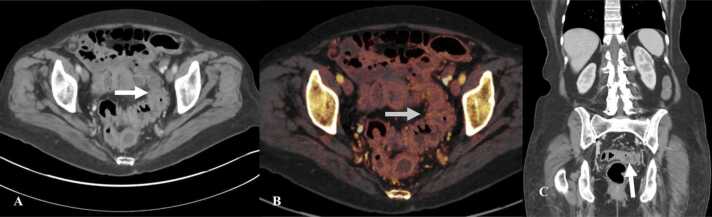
Immune-mediated colitis in a 73-year-old woman with renal cell cancer on Nivolumab. A. Contast-enhanced axial CT of the abdomen and pelvis shows wall thickening and enhancement of the descending and sigmoid colon (arrow) with adjacent fat stranding. B. Color overlay image on dual energy CT shows enhancement in the mucosa of sigmoid colon (arrow). C. Coronal image of the abdomen and pelvis shows wall thickening and enhancement of the mucosa of sigmoid colon (arrow).
